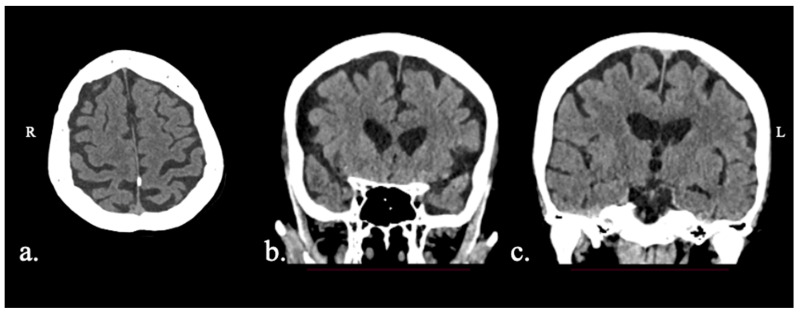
Patient XTY CT scan: (a) axial view with biparietal (L > R) atrophy; (b) coronal view with bilateral symmetric frontal atrophy; (c) coronal view with mild left hippocampal atrophy (Scheltens 1).
